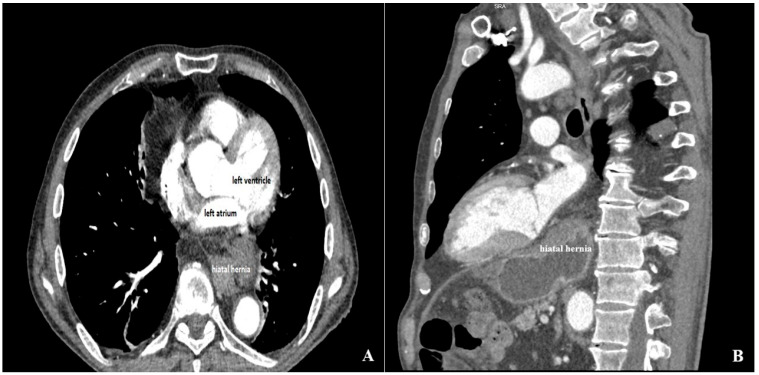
Contrast-enhanced computed tomography showing extracardiac origin. (A) Axial chest CT image showing a hiatal hernia located posterior to the left atrium. (B) Sagittal reconstruction showing posteriorly positioned herniation of the stomach through the esophageal hiatus, adjacent to the left atrium, which confirmed the extracardiac nature of the mass visualized on transthoracic echocardiography.
